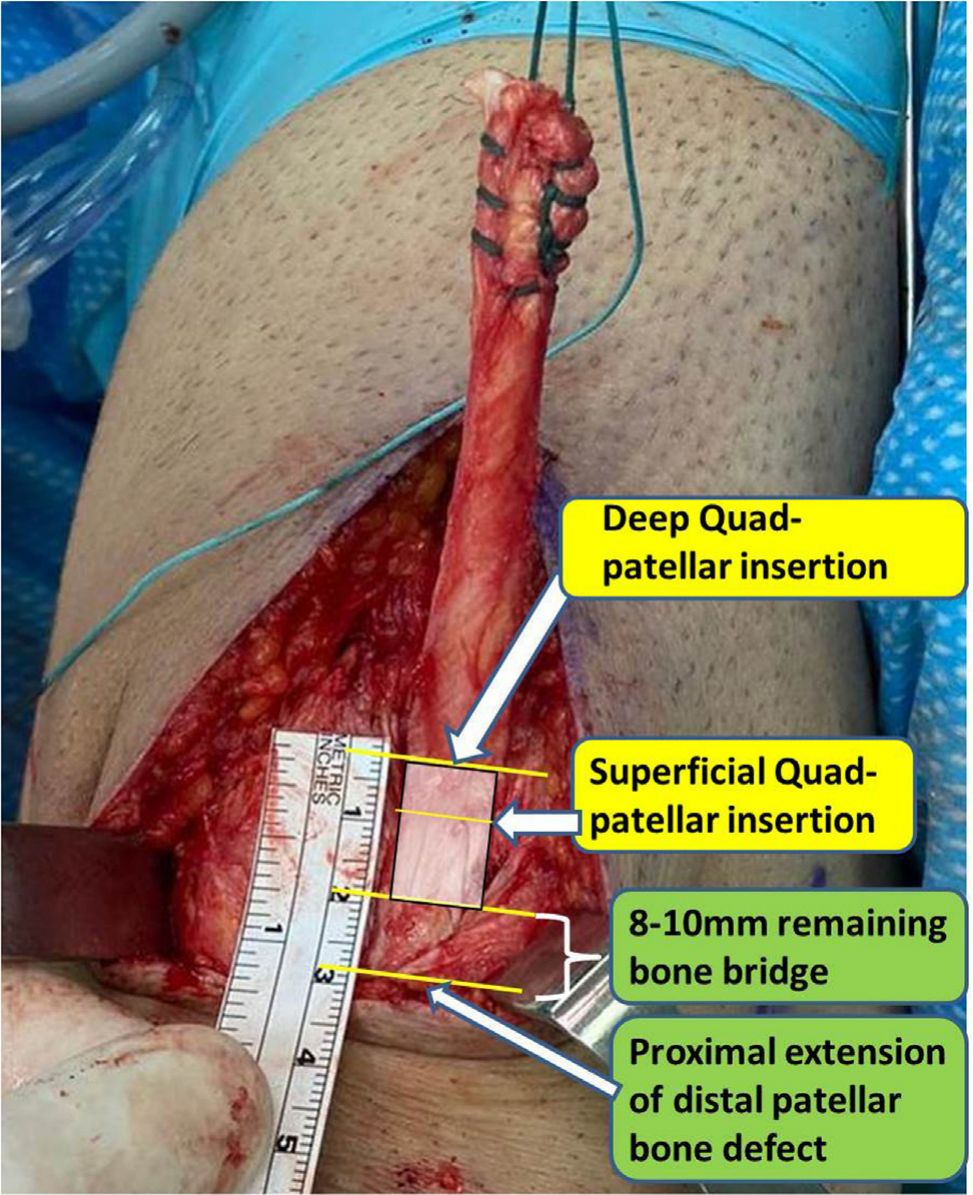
Ipsilateral quadriceps tendon harvest, measuring from 9 to 10 mm wide and 80 to 90 mm long. Harvest is initiated proximally and the tendinous part of the graft is whipstitched and lifted. All reference lines are identified for proper bone plug harvest according to the preoperative computed tomography measurements, including deep and superficial insertions of the quadriceps tendon to the proximal patellar pole, proximal extension of the distal patellar bone defect, and the planned distal extension of the quadriceps–patella bone plug, leaving a bone bridge between the 2 bone plug harvest sites.12.The quadriceps tendon–bone autograft preparation is finalized on a side table. The bone plug is gently tapered with a Rongeur (Aesculap Inc) to smoothly fit the 9.5-mm femoral socket, and the tendinous part should smoothly fit the 10-mm diameter tibial tunnel. Two No. 5 Ethibond sutures (Ethicon) are passed in two 1.5-mm drill holes made in the patellar bone plug (Fig 21).
